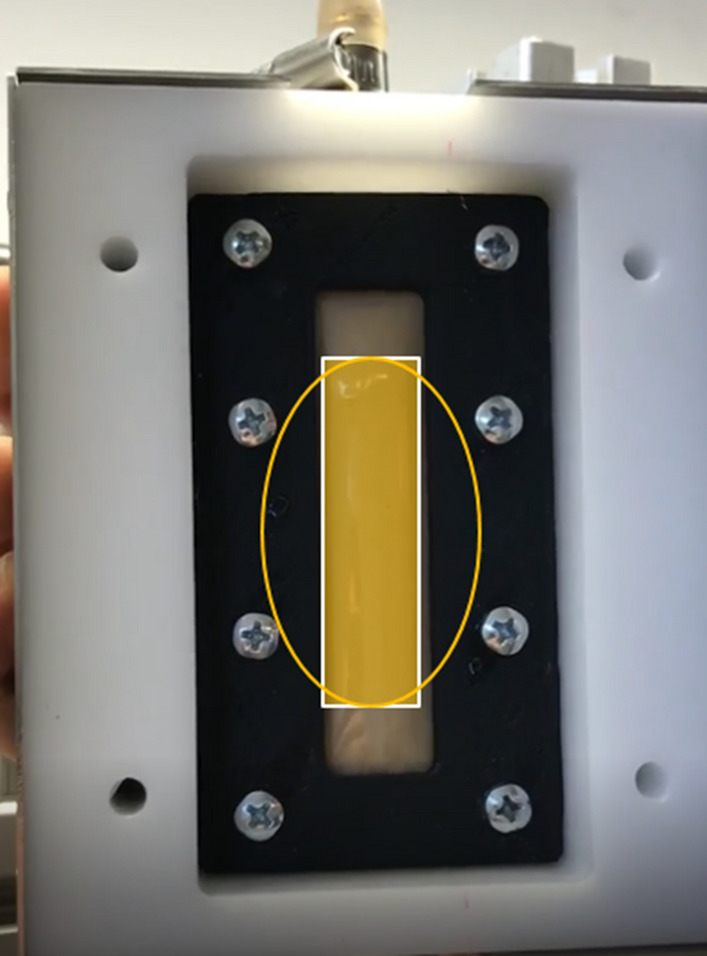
X-ray beam forms an elliptical spot of 52 × 65 mm on the surface of the measurement window, the surface of the irradiated sample is a rectangle of 20 × 65 mm.
